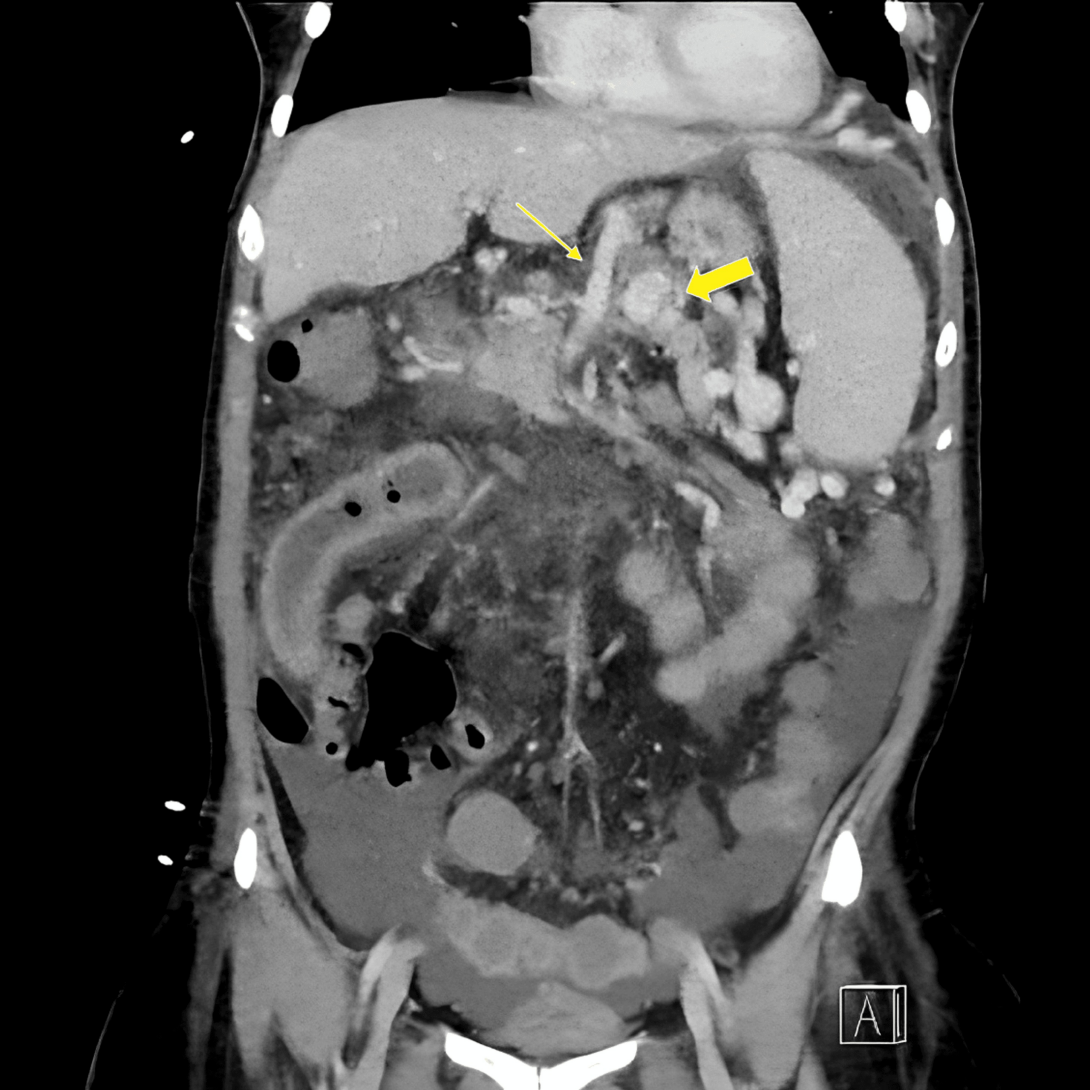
Frontal CT scan showing ascites in the lower abdomen and thickened small bowel loops in the right mid-abdomen. The superior mesenteric vein and splenic vein extend far cranially. The portal vein is absent (Thin arrow: Superior mesenteric vein, thick arrow: Splenic vein).
